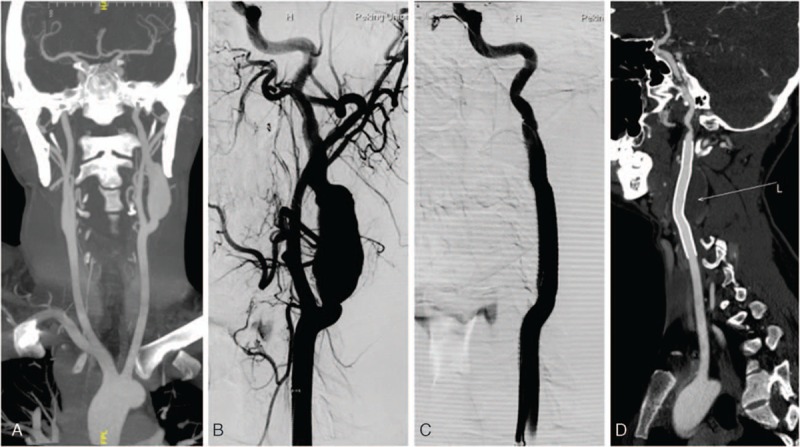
A 24-year-old male presented with a pulsatile mass on his left neck. Preoperative CTA indicated a true aneurysm in the left internal carotid artery (ICA) near the skull base, which is classified as Attigah type II (A). A guide catheter was placed in the ostium of the common carotid artery (CCA). After angiography was carried out through a guide catheter (B), an 8 × 100 mm covered stent (Viabahn, W.L. Gore) was deployed in the segment of the lesion. Immediate angiography showed absolute obliteration of the aneurysm sac with no endoleak (C). Follow-up CTA imaging results at 12 months showed stent patency without restenosis or endoleak (D). CCA = common carotid artery, CTA = computed tomographic angiography, ICA = internal carotid artery.
